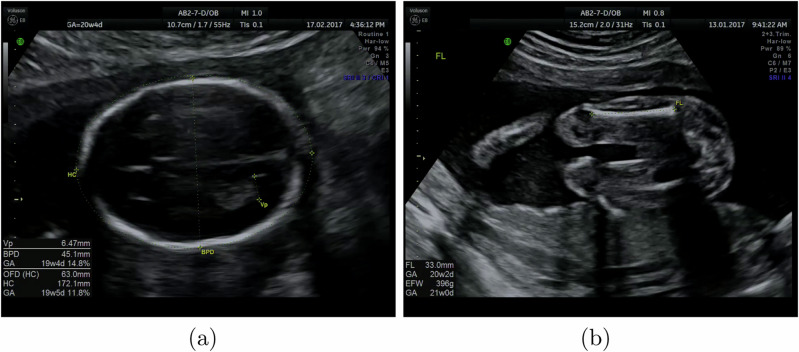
Example frames from routine ultrasound examinations with a sonographer’s annotations and calipers. Shown are a a Brain-TV image, with annotations for head circumference, biparietal diameter and posterior ventricle, b a femur image, with the femur length measured and annotated. These frames were acquired and saved by a sonographer, and we extracted their annotations to form part of our training set.
